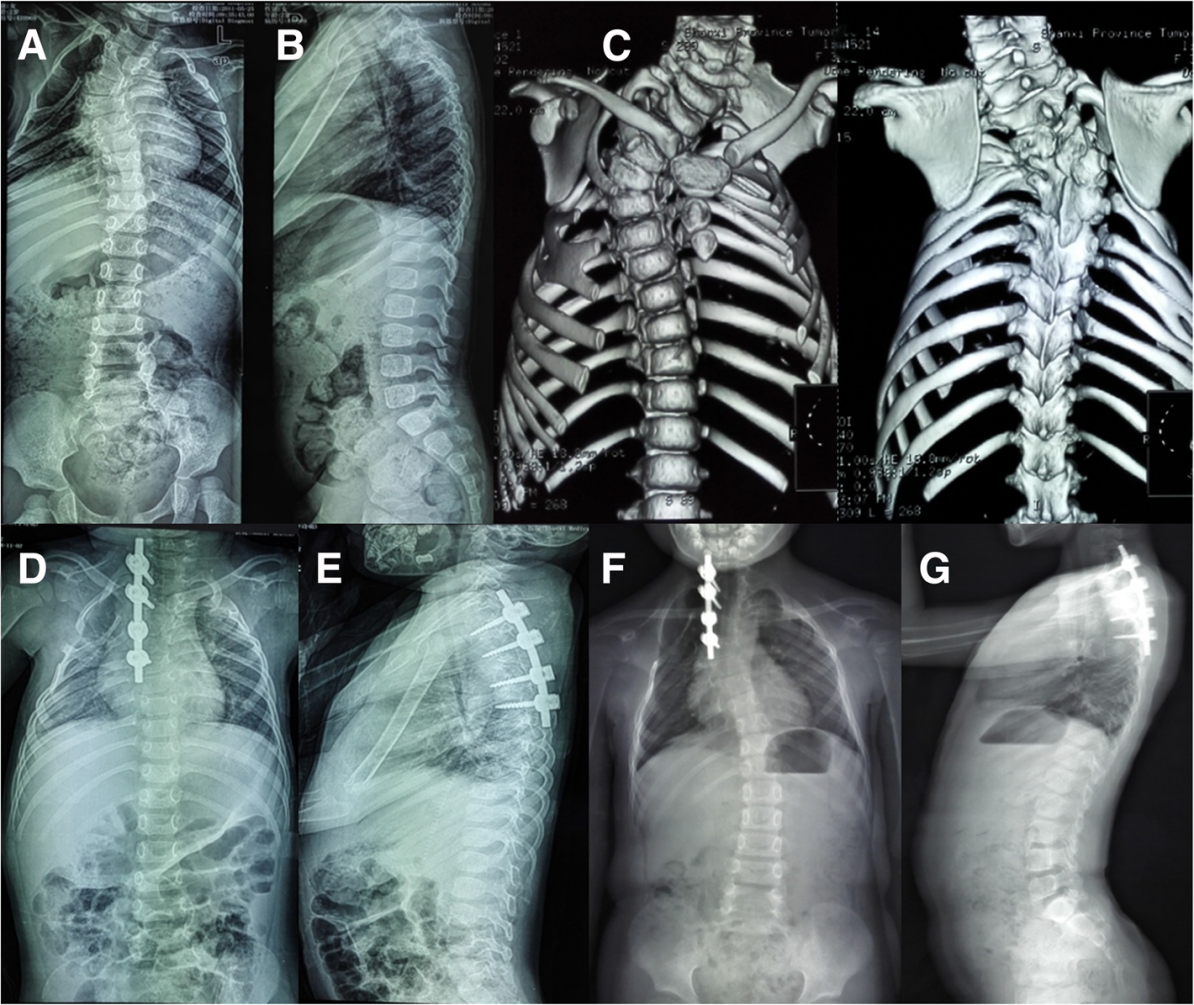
A 2 years and 4 months old girl with congenital scoliosis. Radiographs and 3D-computed tomography images obtained preoperatively (a, b, c), postoperatively (d, e), and at the latest follow-up visit, 60 months later (f, g)
